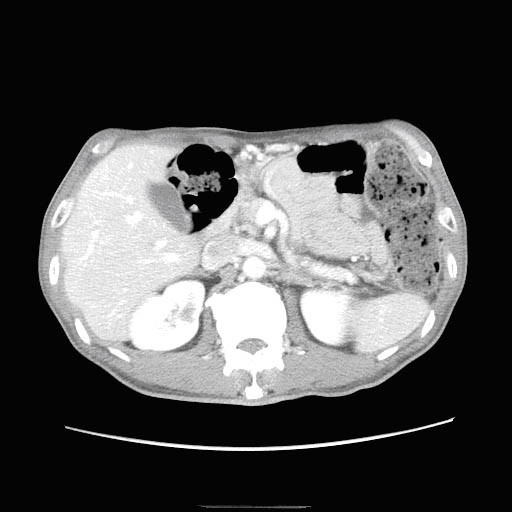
CT showed reduction of the right renal mass, and disappearance of the left renal mass.
